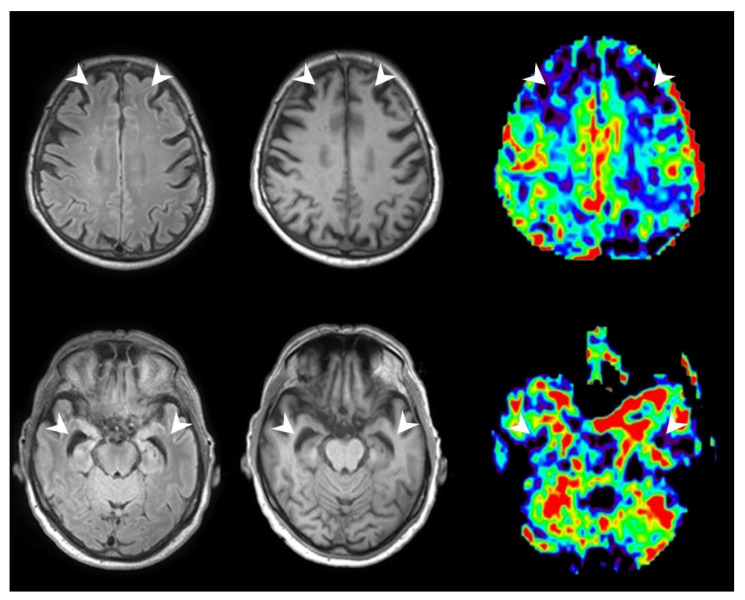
A patient with diagnosed FTD and corresponding pASL perfusion maps showing reduced CBF in the frontotemporal regions. From left to right: FLAIR Sequence, T1w Sequence, pASL Sequence; perfusion data were visualized with OLEA SPHERE^®^ 2.3 (Olea medical, La Ciotat, France)(white arrows indicating regions of atrophy and hypoperfusion).
